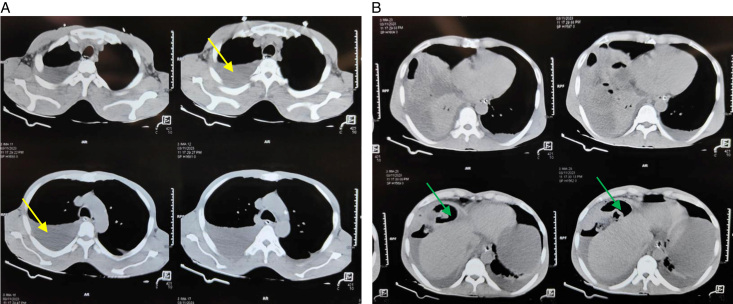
(A) Contrast-enhanced computed tomography (CECT) thorax showing collapsed right lung (yellow arrow). (B) CECT abdomen showing herniated small bowel loops over the liver (green arrow).
